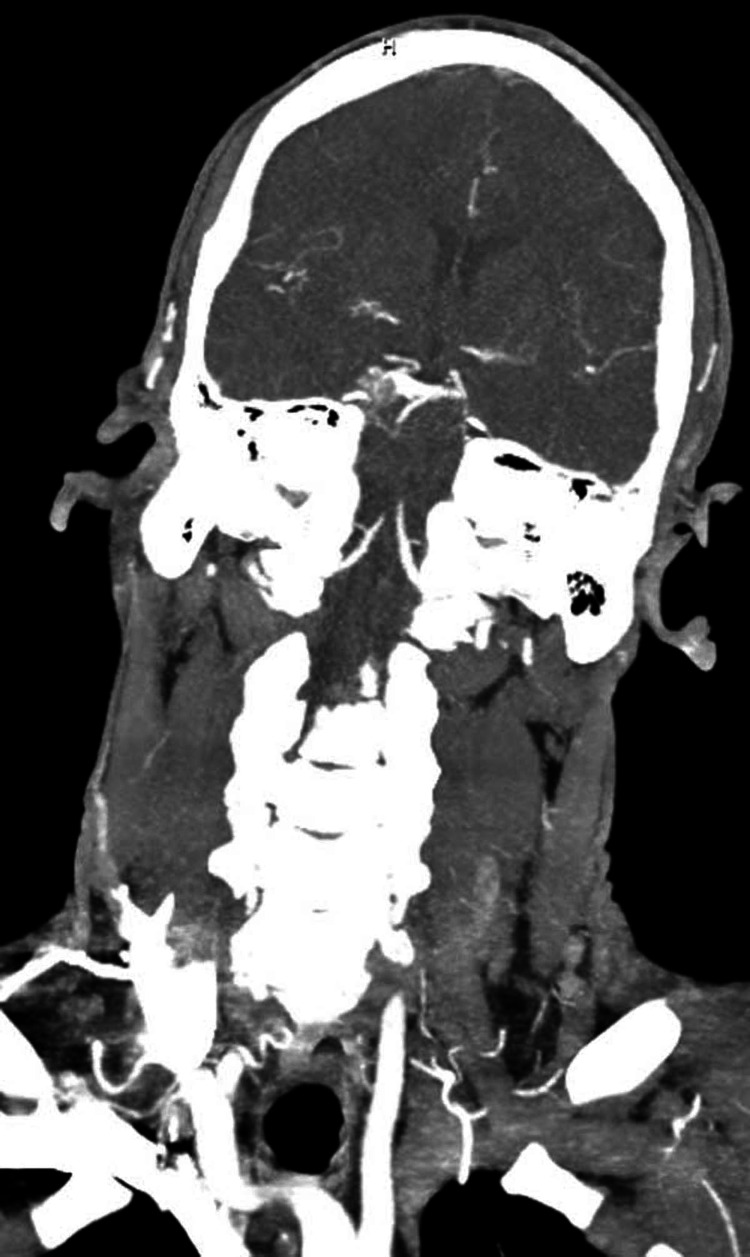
CTA of the head and neck: coronal view. The image shows no large-vessel occlusion, aneurysm, or vascular malformation. CTA = computed tomography angiography
